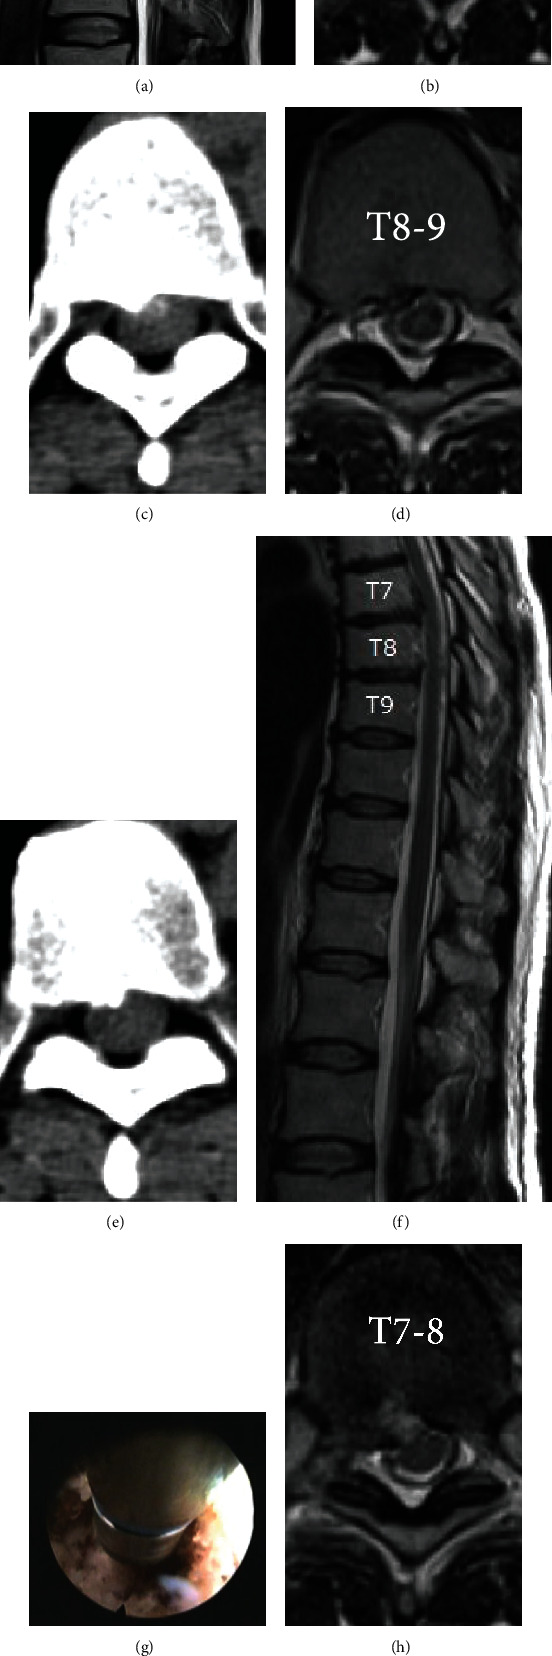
Case presentation of a 49-year-old female, presented with thoracic back pain and gait disturbance for a 3-year duration. Preoperative sagittal (a) and axial (b and d) MRI and CT scan (c and e) showing calcified disc herniation compressing the spinal cord at the T7-8 and T8-9 levels. Transforaminal endoscopic discectomy under local anesthesia was done using a high-speed articulating burr to drill bone spur (g). Postoperative sagittal (f) and axial (h and i) MRI shows full decompression and the patient's pain and myelopathy were improved.
